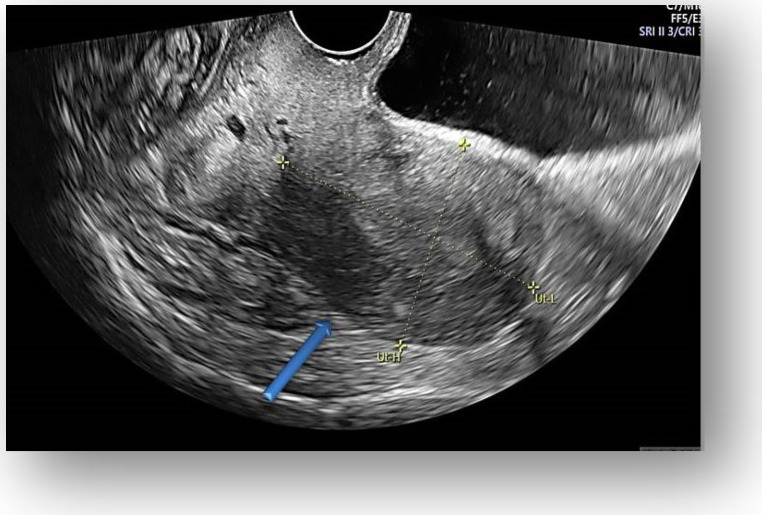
Transvaginal ultrasound showed uterine body with normal endometrium.
